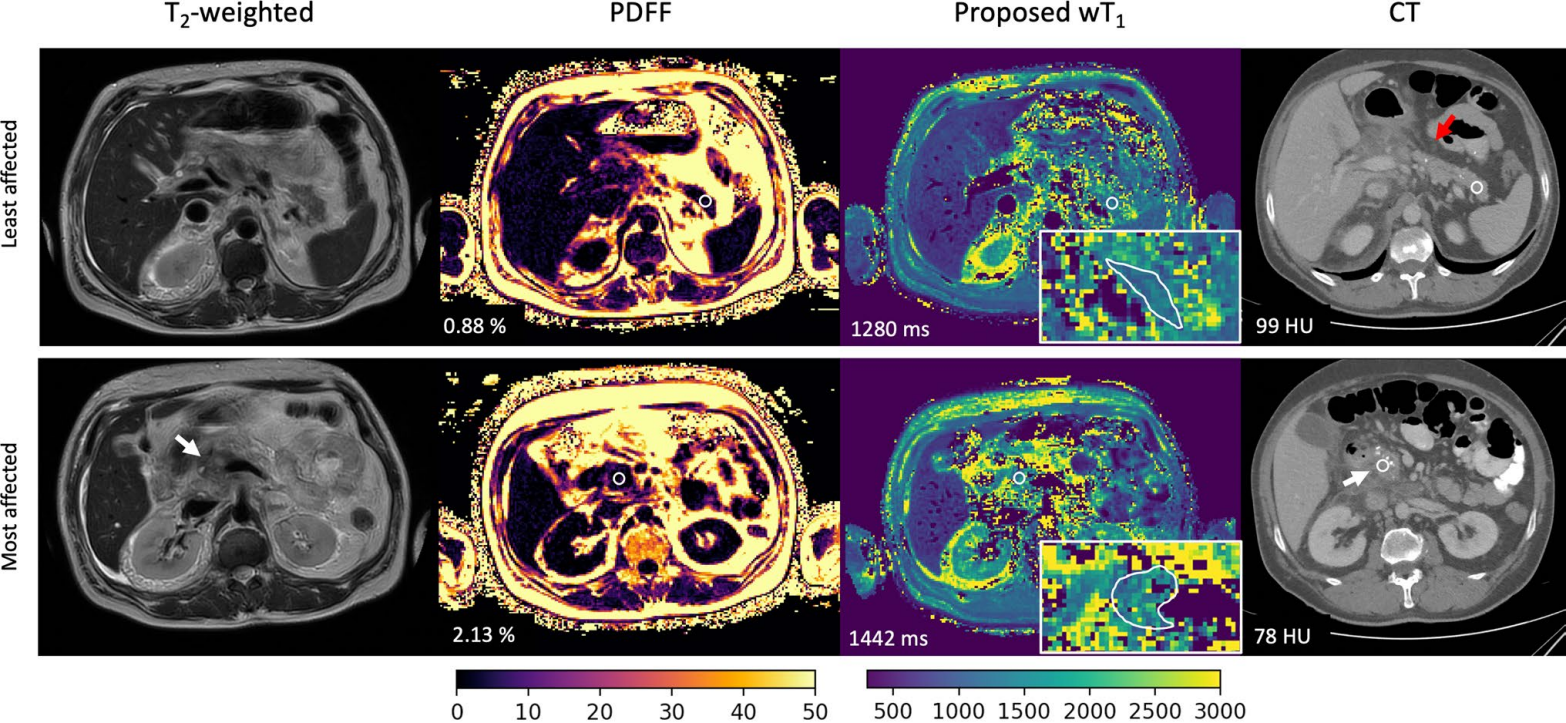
Differentiation of regions affected by pancreatitis in clinical images and wT_1_ maps. A 52-year-old man with a history of alcohol abuse was hospitalized due to necrotizing pancreatitis. T_2_-weighted images, PDFF maps, and CT images were compared to the proposed wT_1_ maps. The CT images, acquired one week earlier during the arterial and venous phases, reveal that the tail of the pancreas (first row) is the region least affected by the pancreatitis, while the head of the pancreas (second row) is the most affected. The images show pancreatic swelling, predominantly in the head (white arrows), characterized by heterogeneous and reduced enhancement, along with fluid collection in the peripancreatic area. Mild peripancreatic fat stranding is also evident (red arrow), particularly near the body and tail of the gland. ROIs (white circles) indicate a higher PDFF value in the most affected areas, confirming the presence of fat within the pancreatic parenchyma. Additionally, the ROIs show that the wT_1_ maps provide differentiation between the least and most affected regions as depicted by the CT Hounsfield unit (HU) values
